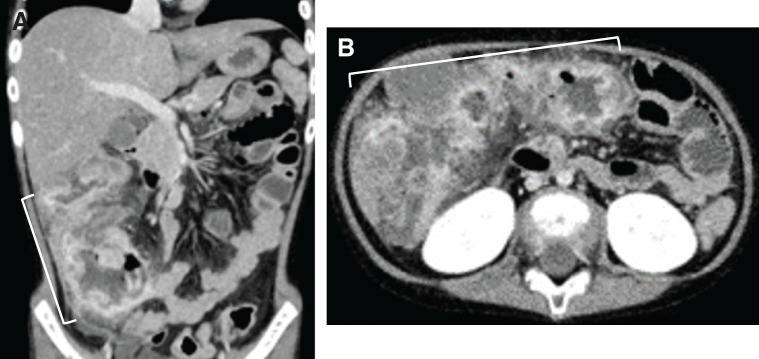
Abdominal CT scans on hospital day 38. On day 38, when the CRP level was markedly elevated, the scans demonstrated mural thickening, intramural abscesses, and increased attenuation of the surrounding fat extending from (A) the ileum to (B) the transverse colon (bracket). CRP, C-reactive protein
